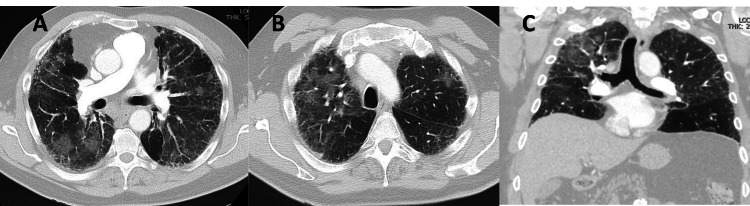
CTA showing ground glass opacities consistent with ILD. A, transverse section of lung (proximal); B, transverse section of lung (distal); C, sagittal section of lung CTA, CT angiograph; ILD, interstitial lung disease
